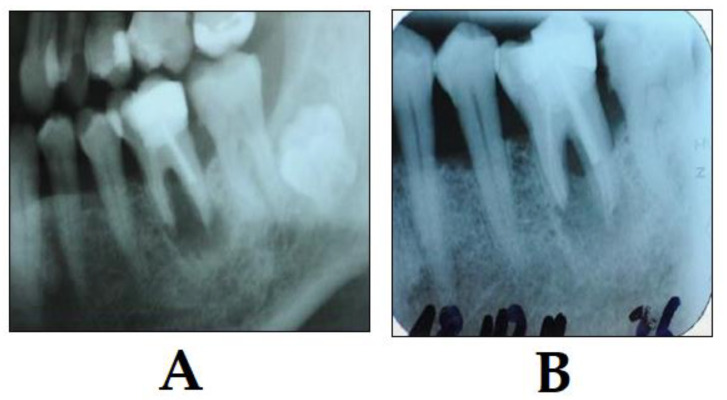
Example of PDT in periodontal therapy (photo courtesy of R. Szabó DMD, J. Arentz DMD and T. Nave DMD). (A) X-ray shows a perio-endo lesion of a tooth before PDT; and (B) X-ray shows the condition three months after PDT. Bone regeneration is visible showing clear signs of clinical improvement.
